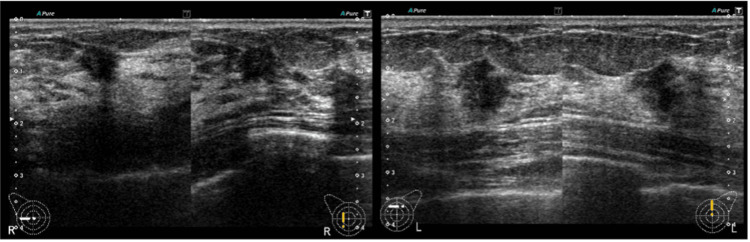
Breast ultrasonographic findings An irregular hypoechoic mass measuring approximately 8 mm in maximum diameter is observed at the 9 o’clock position in the right breast, and an irregular hypoechoic mass measuring approximately 11 mm in maximum diameter is observed at the 12 o’clock position in the left breast
